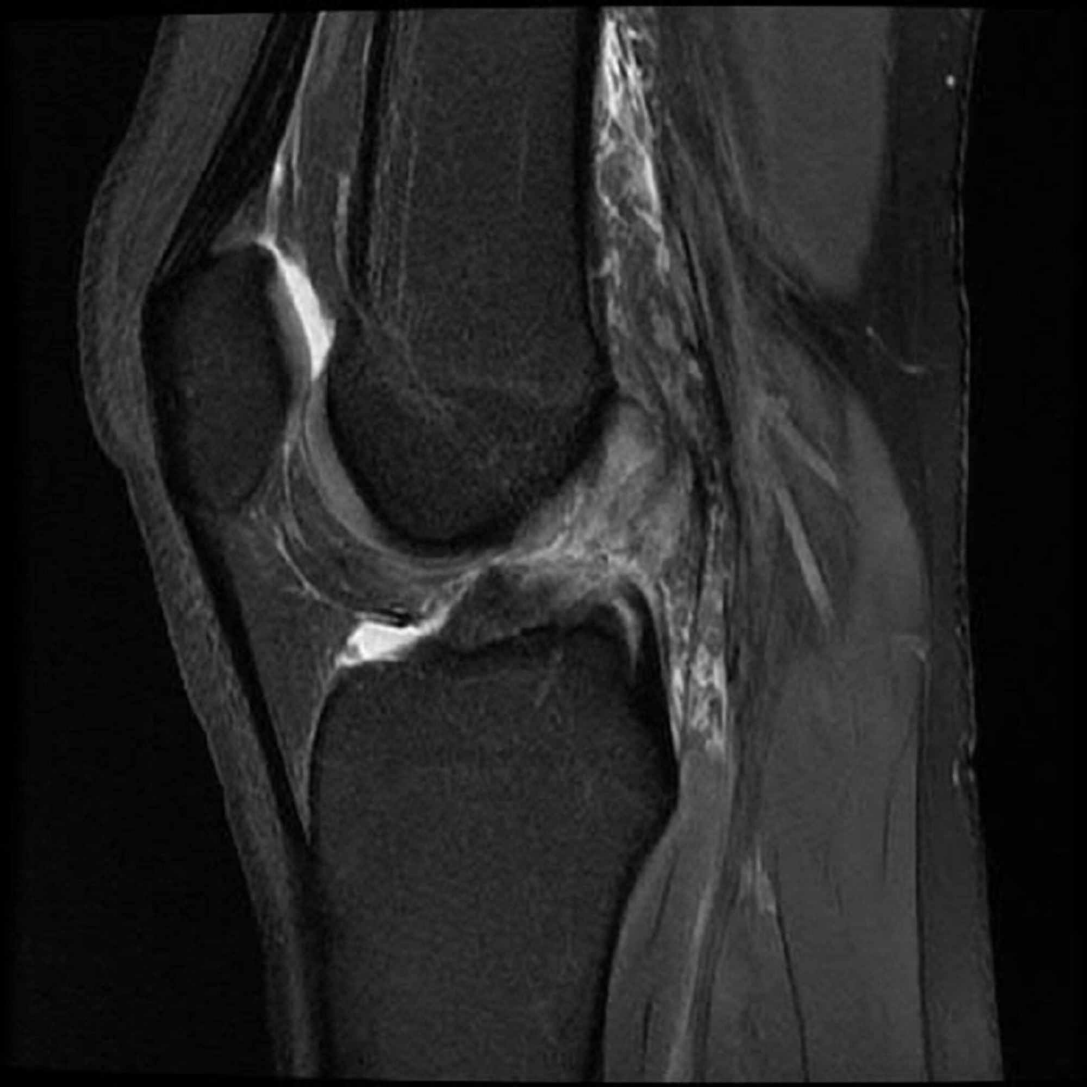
Magnetic resonance imaging (MRI) which showed a complete anterior cruciate ligament (ACL) tear with associated bone contusions in Patient B
